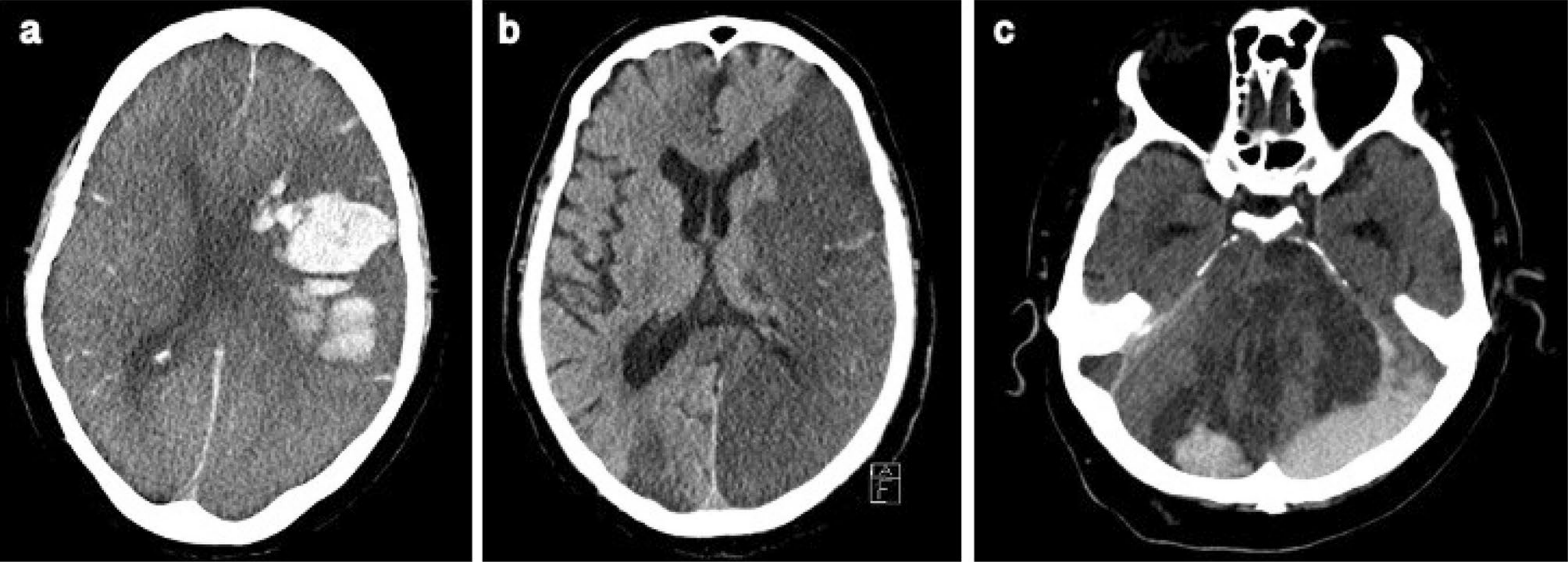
Acute PNIF on Brain CT in three exemplary cases. (a) Case 1. Lobar hemorrhage in the left hemisphere and global subarachnoid hemorrhage with secondary intraventricular hemorrhage. (b) Case 8. Multiple, presumably embolic, infarcts in multiple cerebral circulations (left MCA, right ACA, PCA bilateral, cerebellum). (c) Case 12. Intracranial hemorrhage after multiple infarcts (PCA bilateral, brainstem and cerebellum). CT angiography was not performed in this fatal case but the underlying cause was assumed to be basilar artery occlusion.
